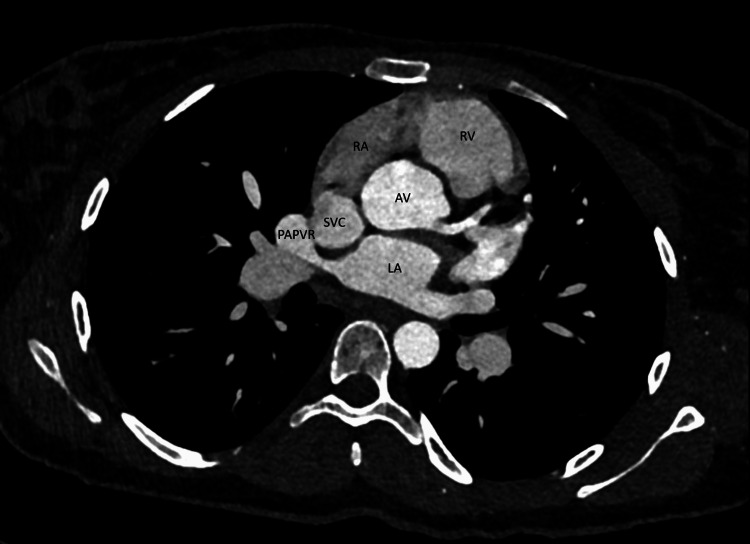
Cardiac CT demonstrating partial anomalous pulmonary venous return (PAPVR) of the right upper pulmonary vein draining into the superior vena cava. Axial image of PAPVR into the superior vena cava (SVC). Relevant adjacent cardiac structures such as the left atrium (LA), right atrium (RA), right ventricle (RV), and aortic valve (AV) are labeled, respectively.
